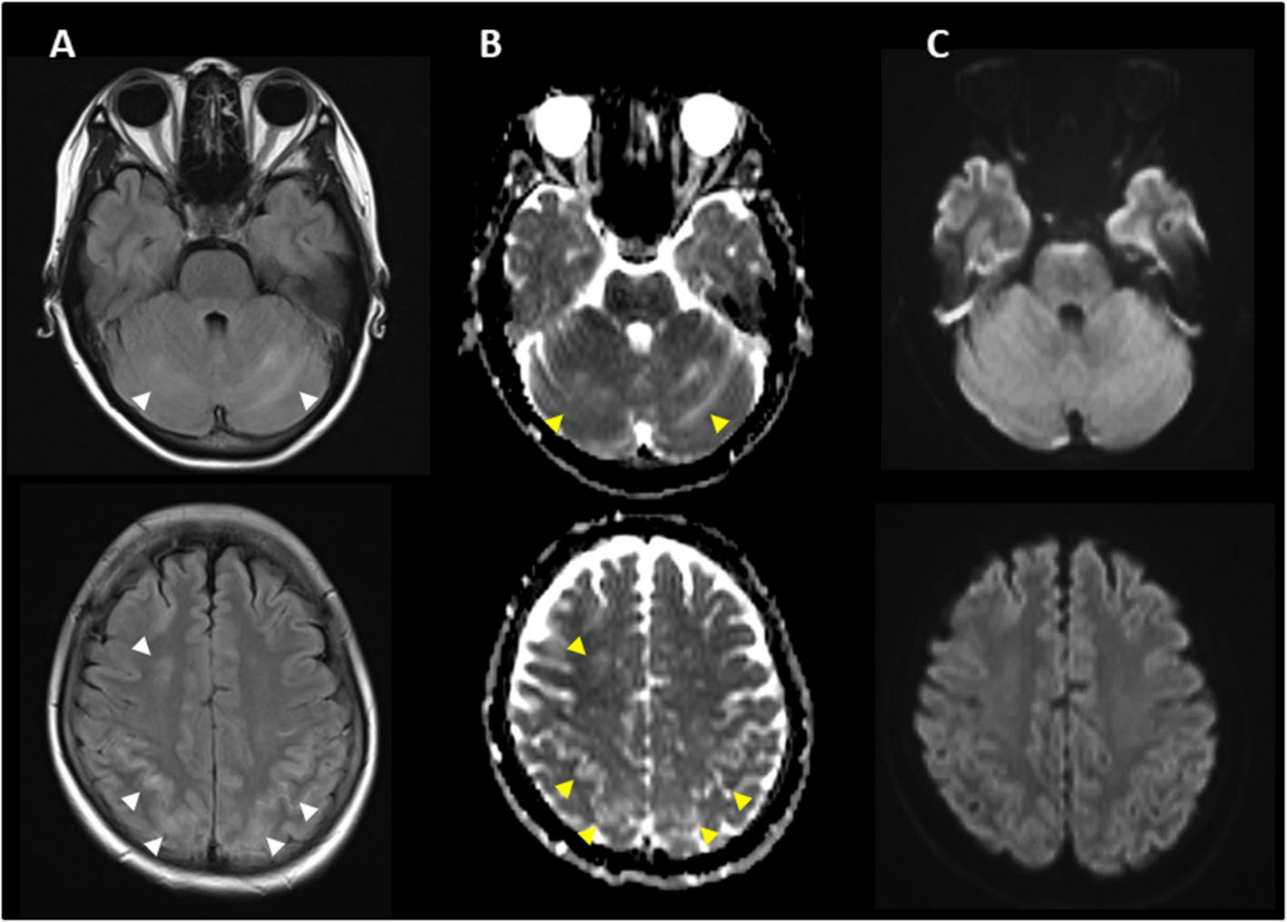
Brain magnetic resonance imaging (MRI) 5 days after onset of first headache. (A) Fluid-attenuated inversion-recovery (FLAIR) images showed hyperintense lesions in the right frontal lobe, bilateral parietal cortex and subcortex, and bilateral cerebellum (white arrow heads). (B) Apparent diffusion coefficient (ADC) map showed elevations in the same regions as the lesions observed (yellow arrow heads). (C) Diffusion-weighted image (DWI) showed no hyperintense regions
